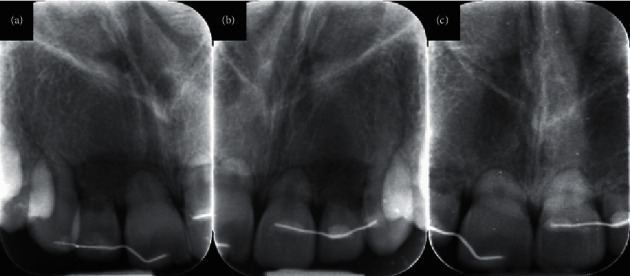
(a) and (b) Periapical radiographs of the upper incisors at 6 months. Slight radiolucent lesions around lateral incisors still persist. (c) Periapical radiograph of the upper incisors at 24 months. Complete healing with no radiolucent area around maxillary lateral incisors.
